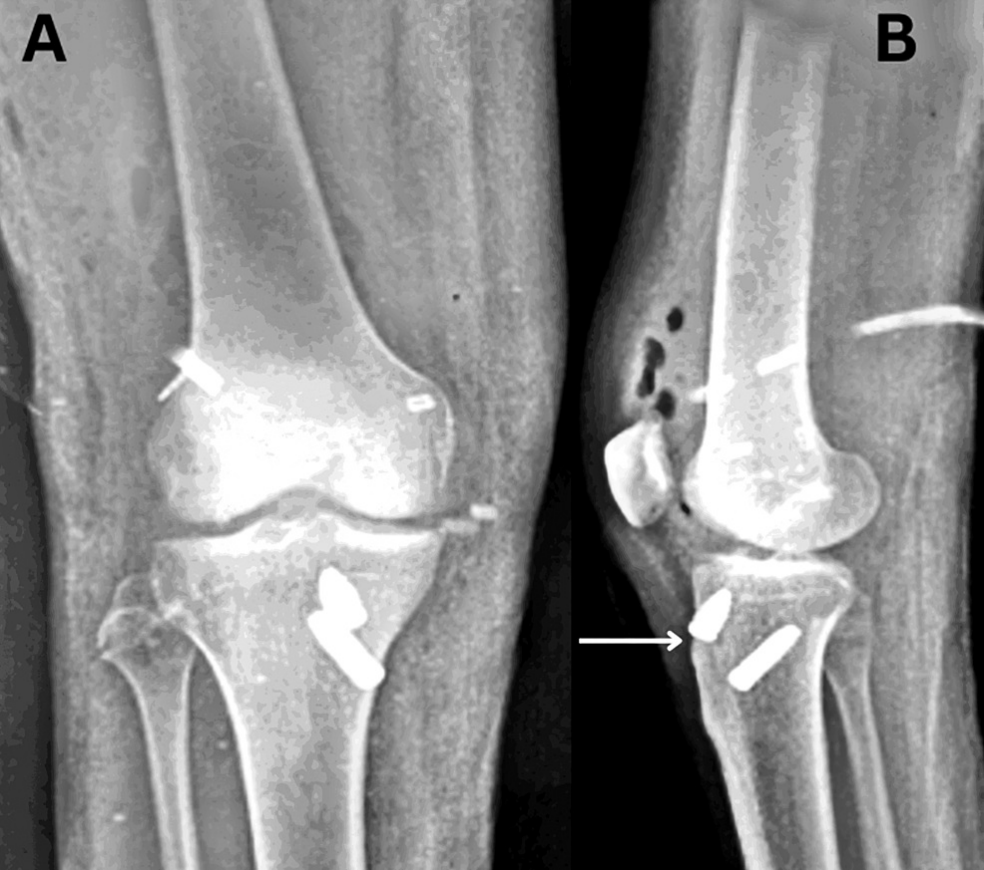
X-ray (A) Posteroanterior view; (B) Lateral view showing arthroscopic reconstruction
